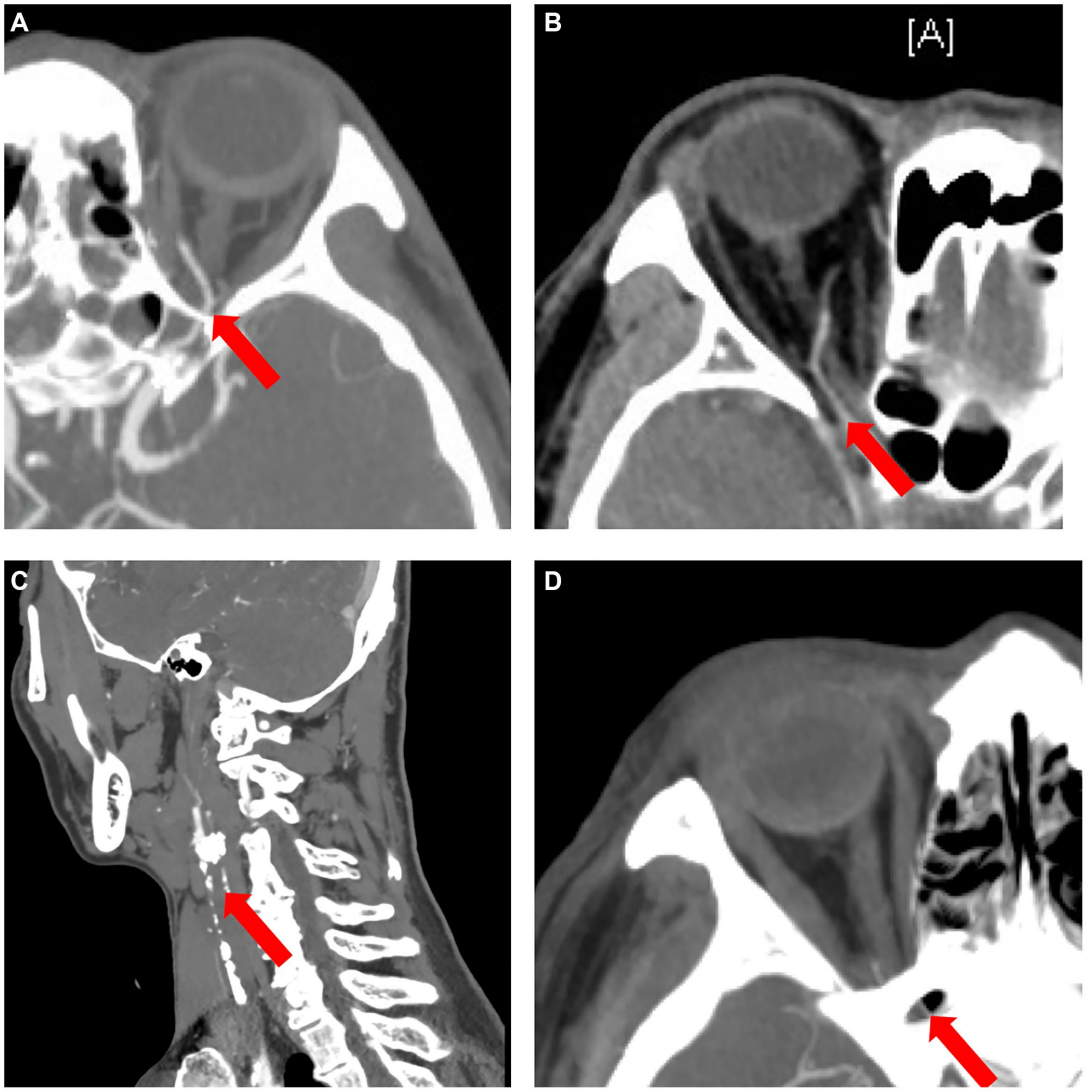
The ophthalmic artery features on CTA. (A) Normal control case presenting with good visualization of the trunk OA (red arrow) of the OA. (B) Type 4 OVA presenting with reversed visualization of blood flow in the trunk OA (red arrow) on DSA from Case No. 6, also showing a narrowed and stiff OA similar to the type 5 OVA on CTA. (C) Another type 4 OVA showing complete ICAO (red arrow) on CTA in Case No. 11. (D) Type 3 OVA presenting with no visualization of the distal trunk OA (red arrow) on DSA, also showing no visualization of the OA on CTA in Case No. 14. CTA, computed tomographic angiography; DSA, digital subtraction angiography; ICAO, internal carotid artery occlusion; OA, ophthalmic artery; OVA, ocular vascular accident.
